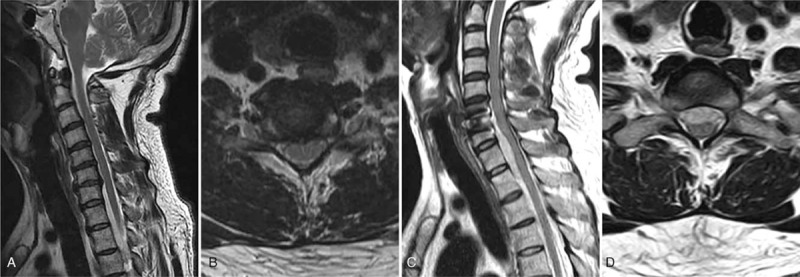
A 42-year-old man presented with CSR (case 1). (A, B) Sagittal and axial MRI before the initial operation showed cervical degeneration, and the C6/7 intervertebral disc was protruding on the right side; (C, D) Sagittal and axial MRI showed epidural hematoma at the C6-thoracic2 (T2) level behind the vertebrae after the ACDF surgery.
